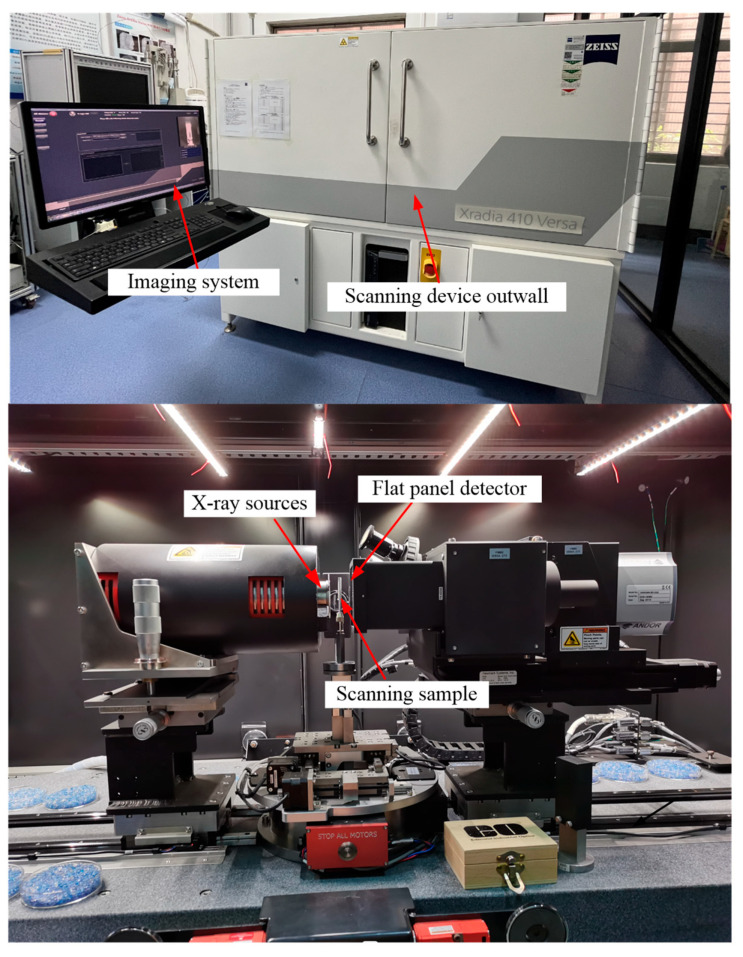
CT scanning imaging system and internal structure.
